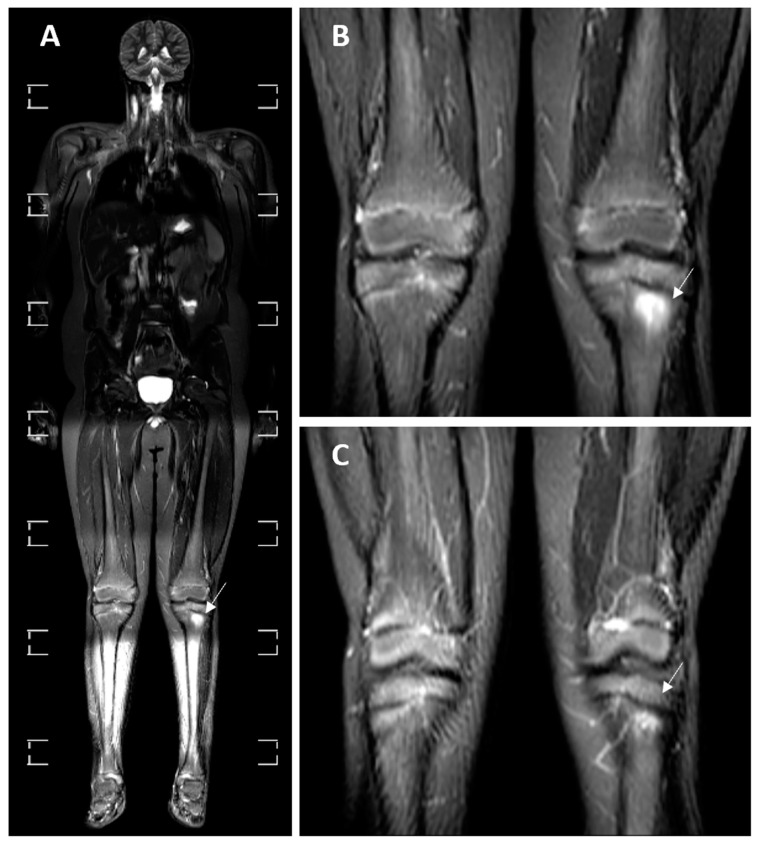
Whole-body MRI in the STIR sequence of an 11-year-old male patient with CNO and a history of joint pain. (A) A whole-body MRI scan. (B,C) Two consecutive cross-sections of the knee joints. The signal elevation in the left tibia in the epiphyseal and metaepiphyseal zones is notable, pointed by white arrows (A–C).
